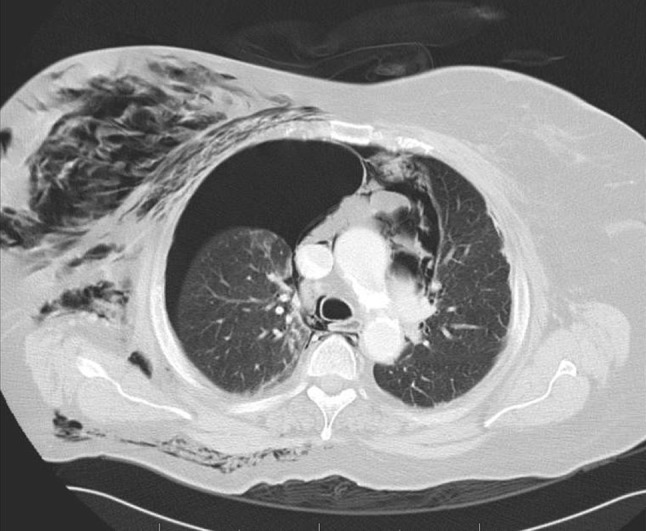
Case 1* Thoracic CT showing right-sided tension pneumothorax with mediastinal shift to the left side. There is a smaller right-sided pneumothorax and also presence of mediastinal, soft-tissue and subcutaneous air
